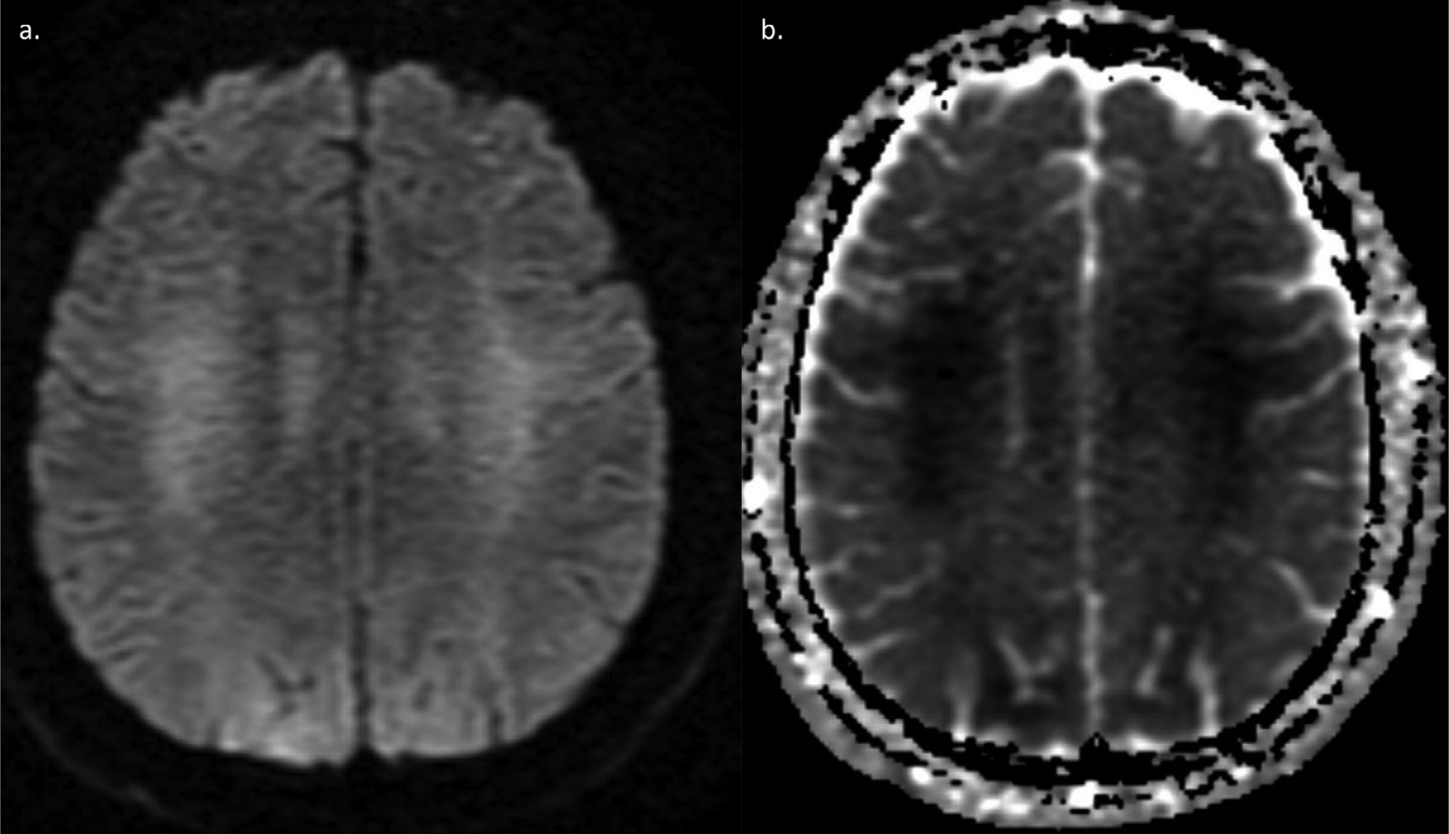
DW-MRI sequence (a) shows increased signal in bilateral centrum semi ovale and posterior parietal cortex and subcortical white matter. Corresponding ADC map (b) shows low signal in the same regions confirming diffusion restriction.
